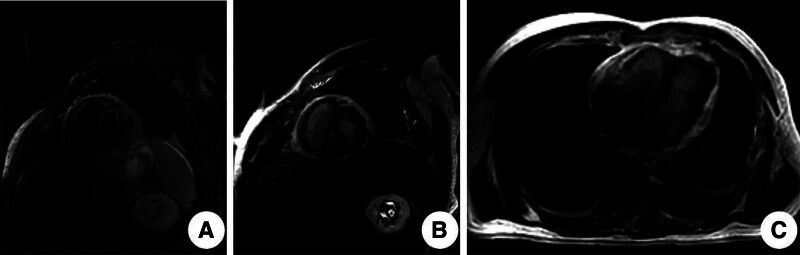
Pretreatment cardiac magnetic resonance (CMR) imaging. (A) T2-weighted short-axis image shows pericardial thickening, with the thickest area measuring approximately 1.2 cm. (B, C) Late gadolinium enhancement (LGE) sequences in short-axis and 4-chamber views demonstrate pericardial enhancement, consistent with active pericarditis.
